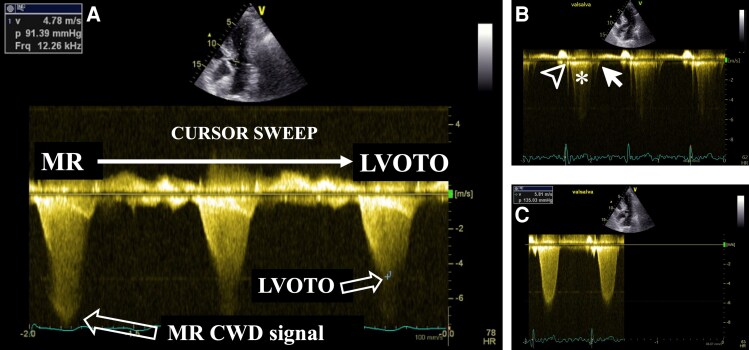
Mitral regurgitation and left ventricular outflow tract Doppler signal. The MR signal has an initial slower acceleration followed by a typical steep–straight acceleration phase (Panel *A*, left signal). The MR signal can be excluded by sweeping the cursor towards the LVOT, resulting in the more late-peaking LVOTO dagger-shaped signal (right signal). In B, the arrowhead and arrow show the start and end of the MR signal, respectively, with part of the LVOTO signal indicated by an asterisk. In C, the MR signal is excluded from the LVOTO signal when sweeping more towards the LVOT.
